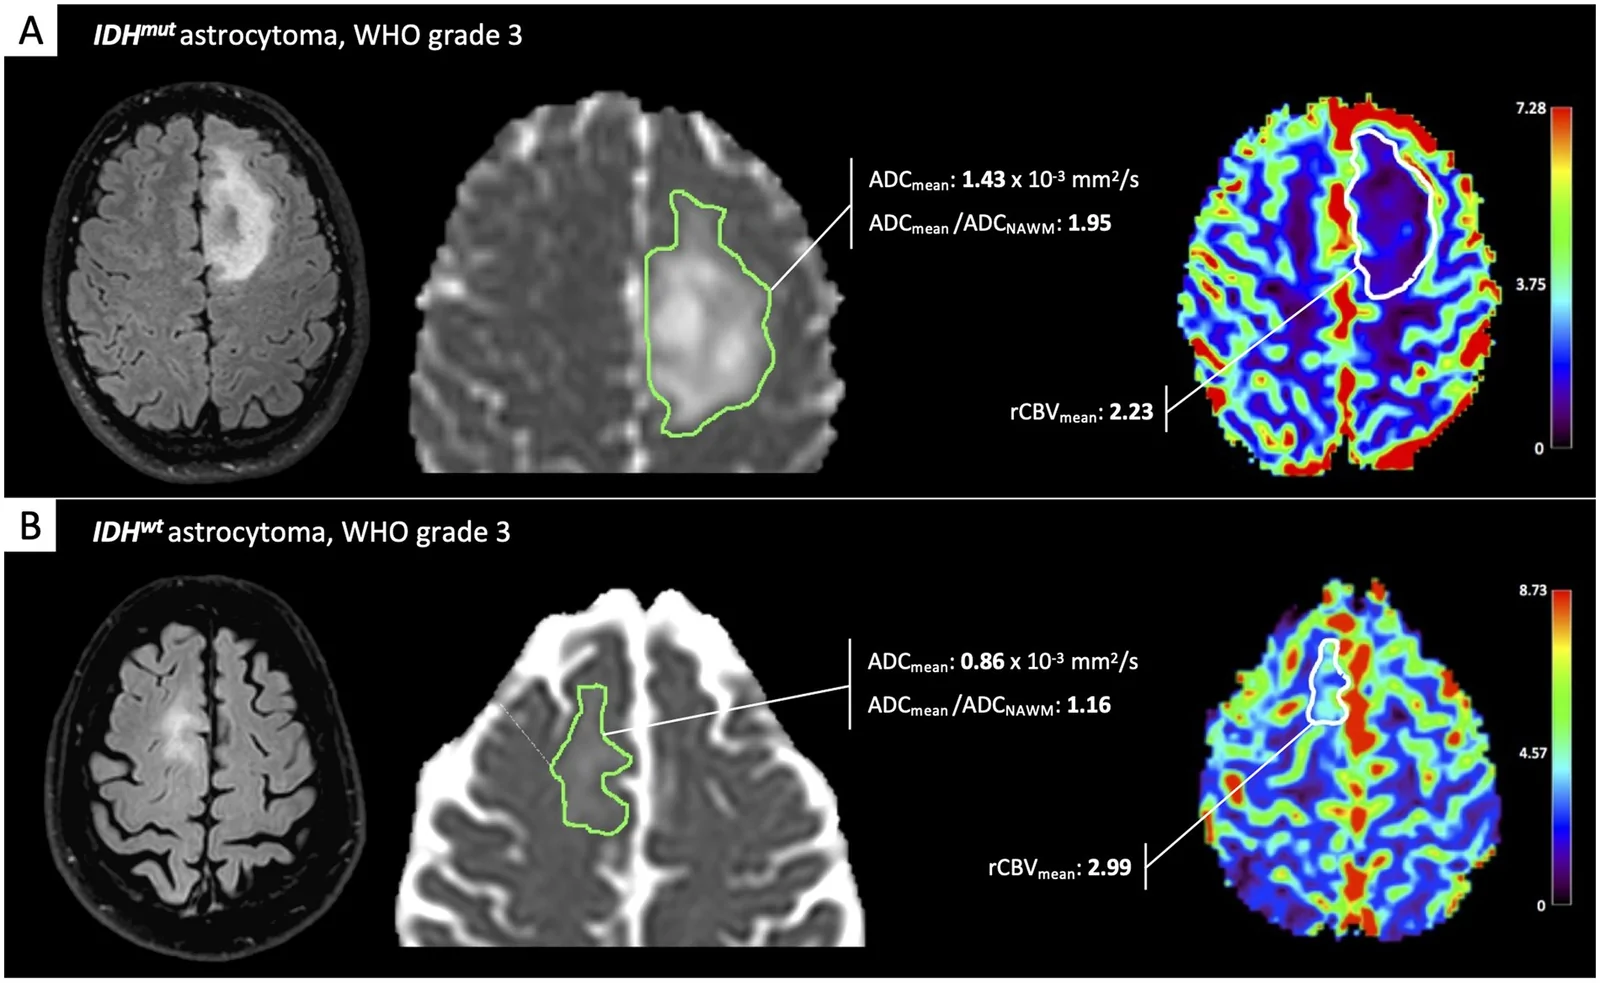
Perfusion and diffusion signatures of IDH mutation in lower grade gliomas. DWI and PWI features from 1 isocitrate dehydrogenase mutant (IDHmut)-LGG (A) and 1 IDH wild type (IDHwt)-LGG (B) were evaluated through a clinically feasible single-slice region of interest (ROI) approach. In contrast to IDH-wildtype LGG, IDH-mutant LGG demonstrates less “aggressive” advanced MRI characteristics, including higher mean ADC/normal-appearing white matter ADC ratio (ADCmean/ADCNAWM) and lower rCBV values. Reprinted from Sanvito et al.,^43^ under the Creative Commons CC BY 4.0 license. Abbreviations: ADC, apparent diffusion coefficient; DWI, diffusion-weighted imaging; LGG, lower grade glioma; PWI, lower perfusion MRI; rCBV, relative cerebral blood volume.
